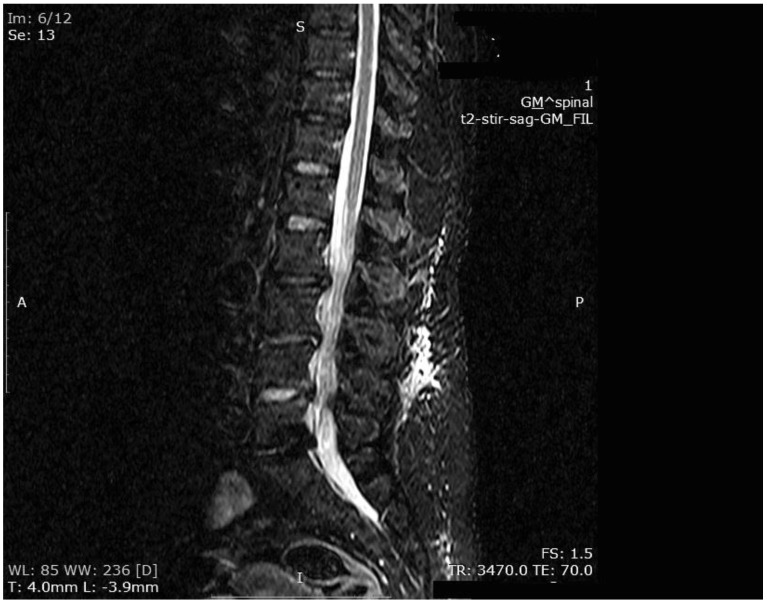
MR imaging scan showing inflammatory infiltrates at the end of HBO_2_ treatment.
